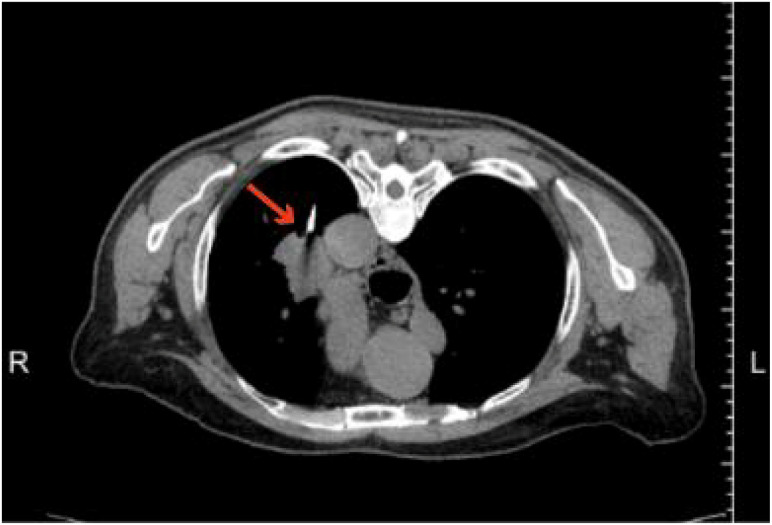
Percutaneous needle biopsy. Under CT guidance, a puncture was performed. The red arrow in figure indicates the puncture needle.
